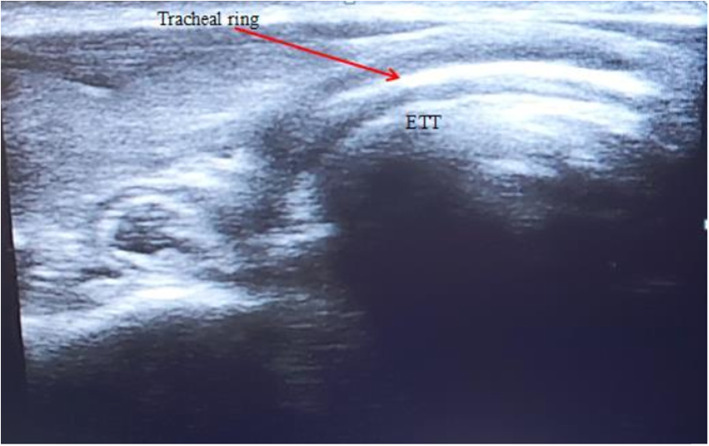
Midline ultrasonographic view of the trachea with the endotracheal tube (ETT) in the right position
